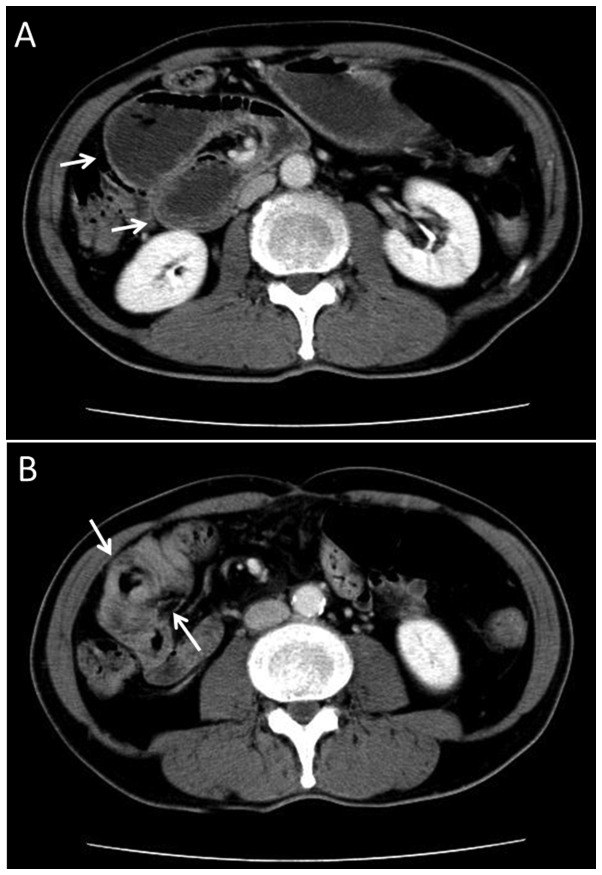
Abdominal contrast CT scan of the patient showing (A) dilatation of the duodenum and the upper side of the jejunum (arrows) and (B) a tumor in the upper side of the jejunum. The tumor was present in almost the entire circumference of the jejunal wall (arrows). CT, computed tomography.
